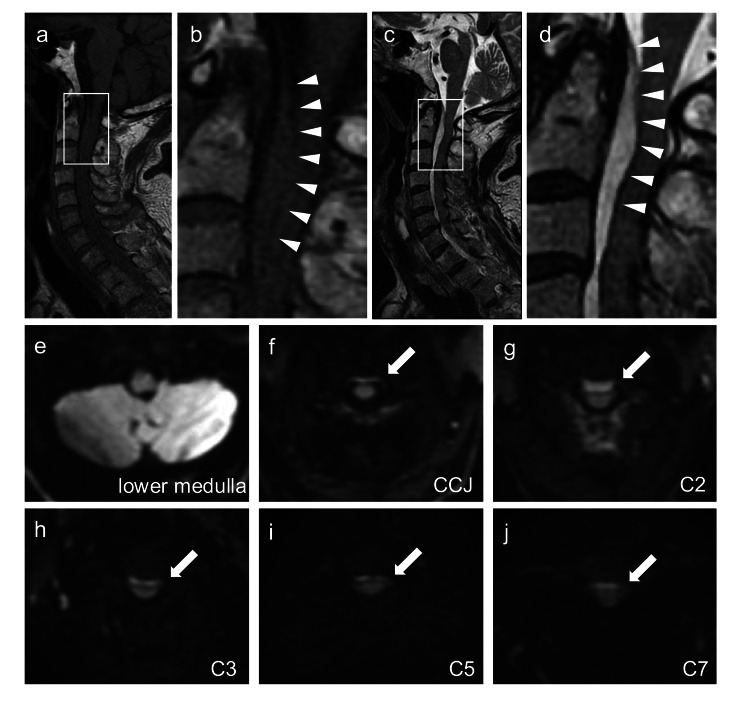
Spine magnetic resonance imaging findings 90 minutes after arrival a, b: Sagittal T1-weighted images; c, d: Sagittal T2-weighted images; e-j: Axial diffusion-weighted images (DWI; b=650 s/mm2) at the lower medulla, craniocervical junction, C2, C3, C5, and C7 levels. Enlarged views of the regions outlined by squares in panels a and c are shown in panels b and d, respectively. Arrowheads indicate a hematoma located ventral to the spinal cord, with posterior compression of the spinal cord observed near the C2 level. DWI shows no abnormality at the lower medullary level but reveals a hyperintense signal, consistent with a hematoma ventral to the cervical cord (arrows). The high-intensity signal is most prominent at the C2 level and extends from the craniocervical junction to the C7 level.
